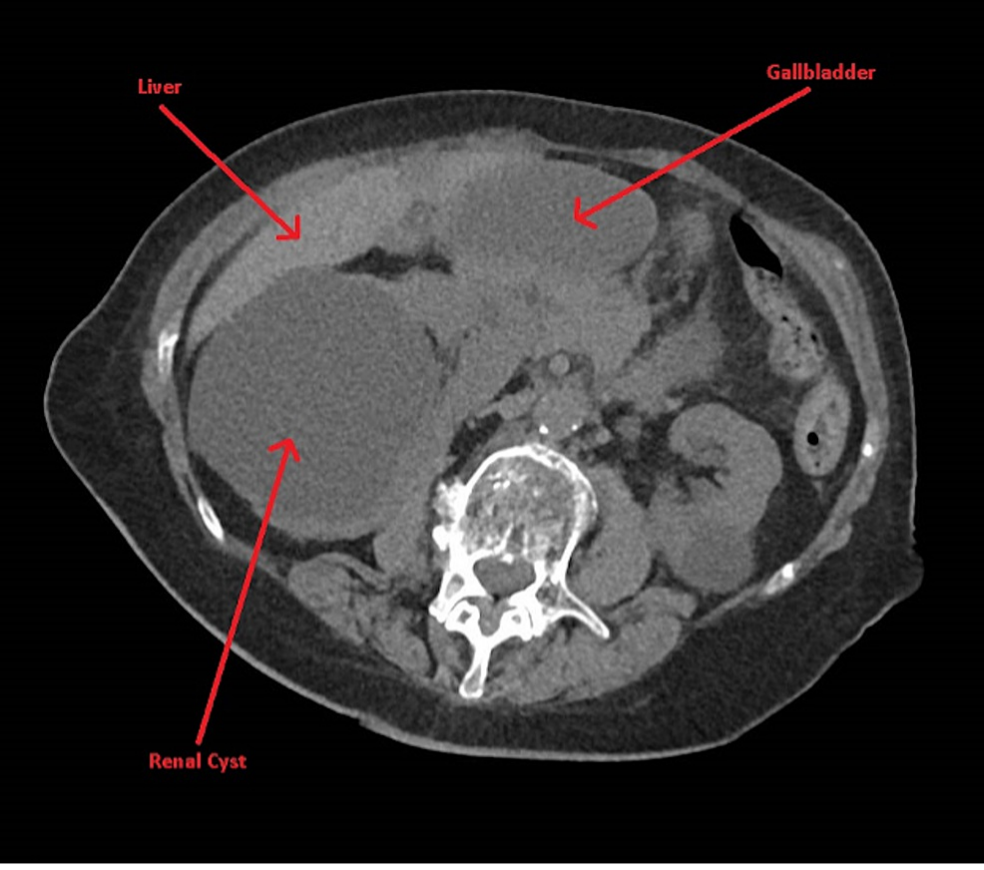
CT Abdomen and Pelvis Axial CT image of the abdomen performed without intravenous contrast demonstrates a distended, horizontally positioned gallbladder. The actual twist in the gallbladder neck is not well seen. There is no pericholecystic inflammation and no appreciable gallbladder wall thickening.
